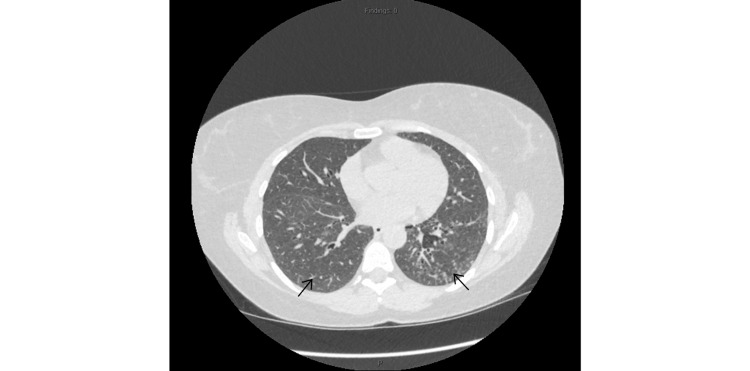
High resolution CT chest- Mild interval improvement in the ground glass nodules and septal thickening (arrows). Follow-up high-resolution CT chest showed interval improvement but persistent bilateral ground-glass opacities with basal interstitial thickening
